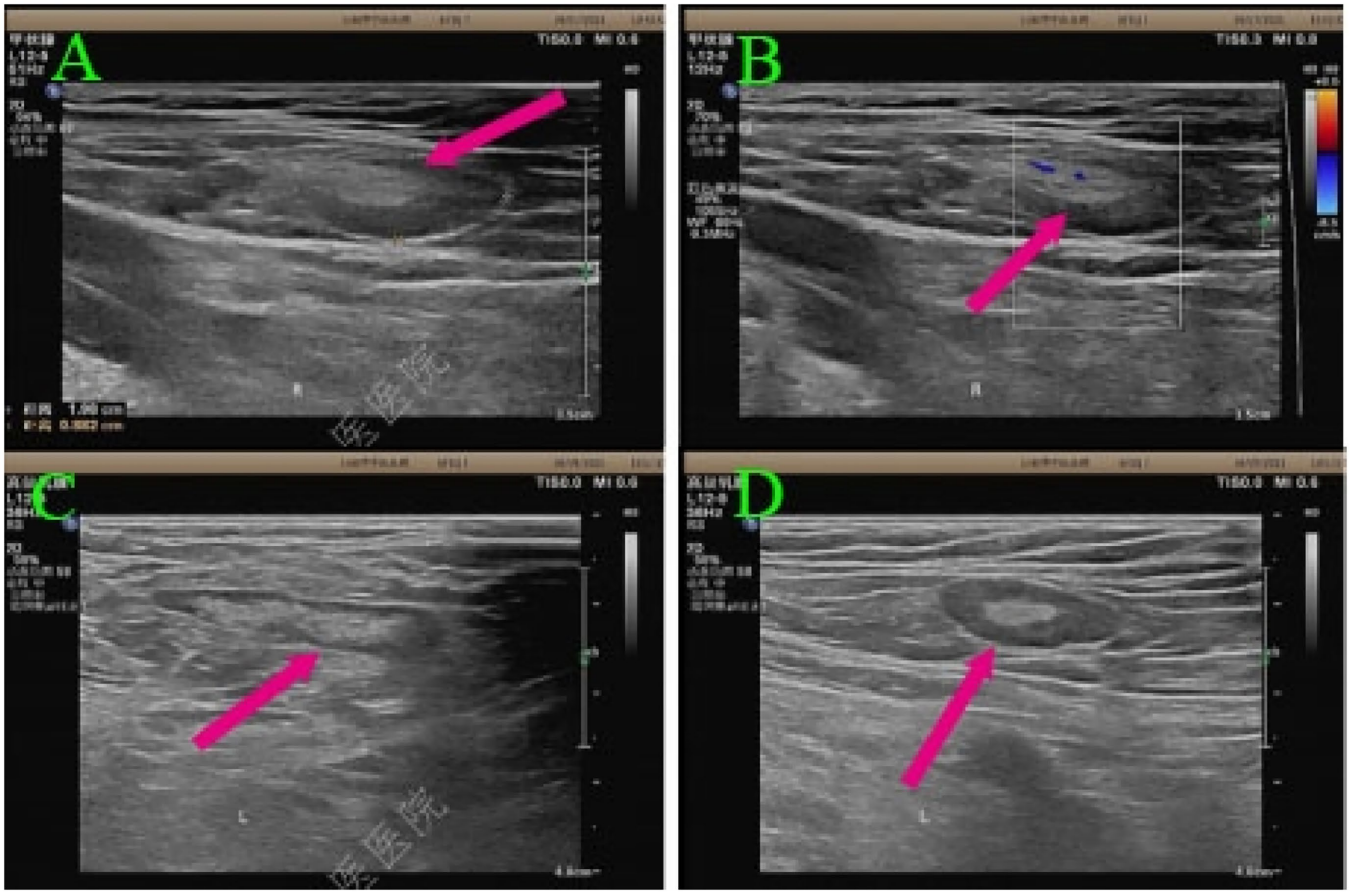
Ultrasonography reveals enlarged lymph nodes in the cervical (A), axillary (B), and inguinal (C,D) regions, with preserved nodal architecture, characterized by regular morphology and visible echogenic hilum, with spot-like and linear blood flow signals within the lymph nodes.
